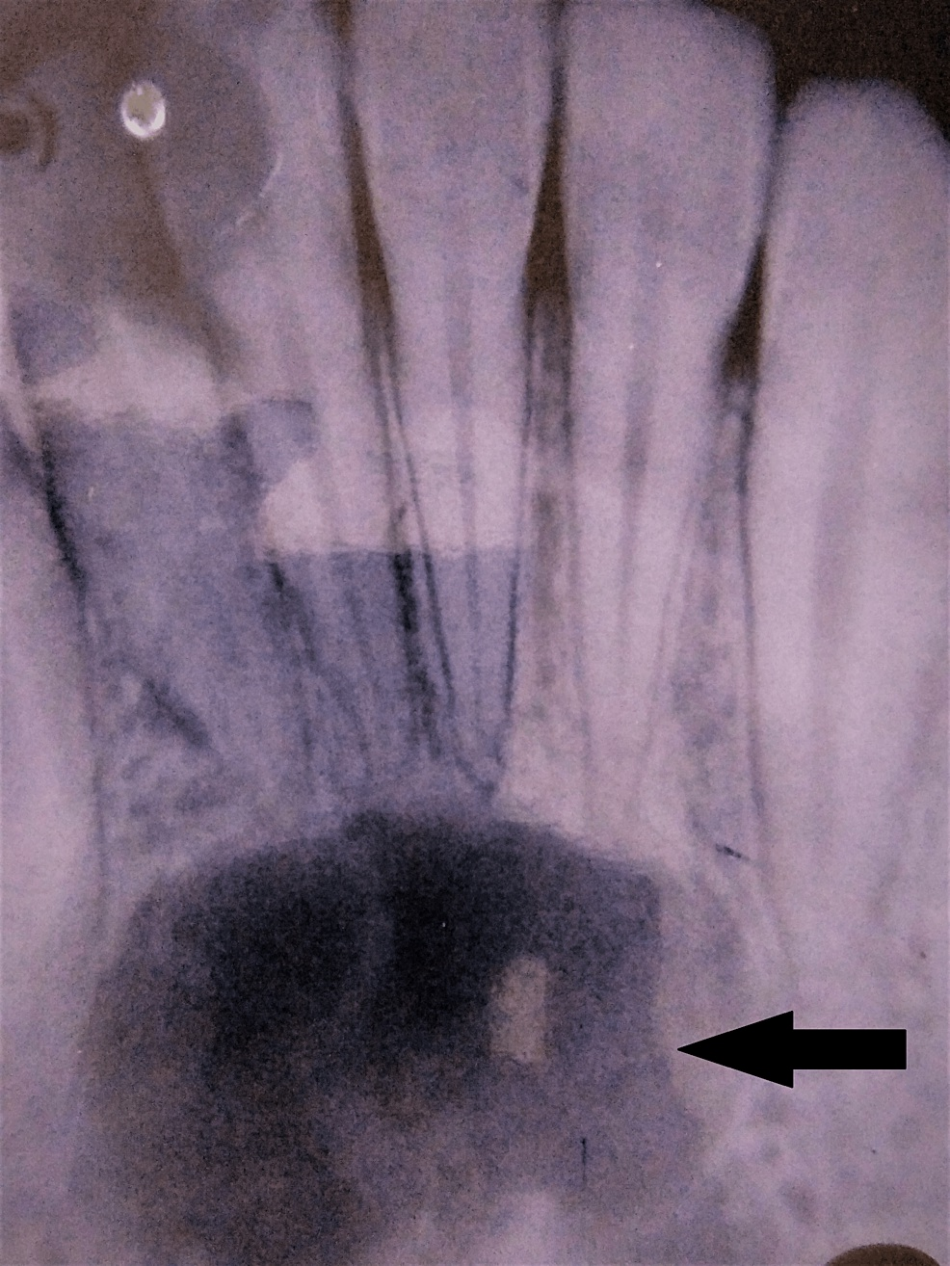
Cyst shown in IOPA radiograph IOPA: intraoral periapical
